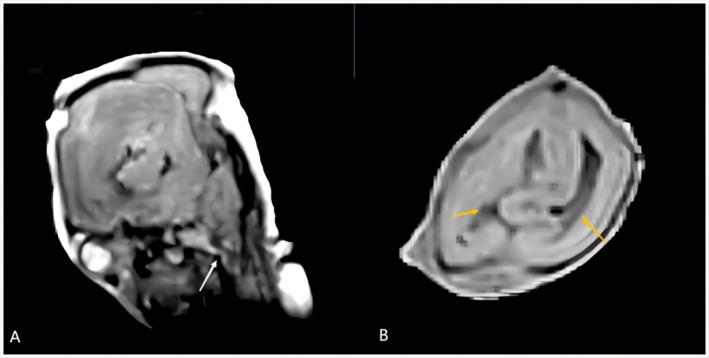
Postmortem MRI findings at 21 weeks and 2 days of gestation. (A) Postmortem MRI reveals cerebellar vermis hypoplasia and a kinked brainstem, indicated by the white arrow. (B) Axial postmortem MRI shows severe hydrocephalus of the lateral ventricles, indicated by the yellow arrows.
